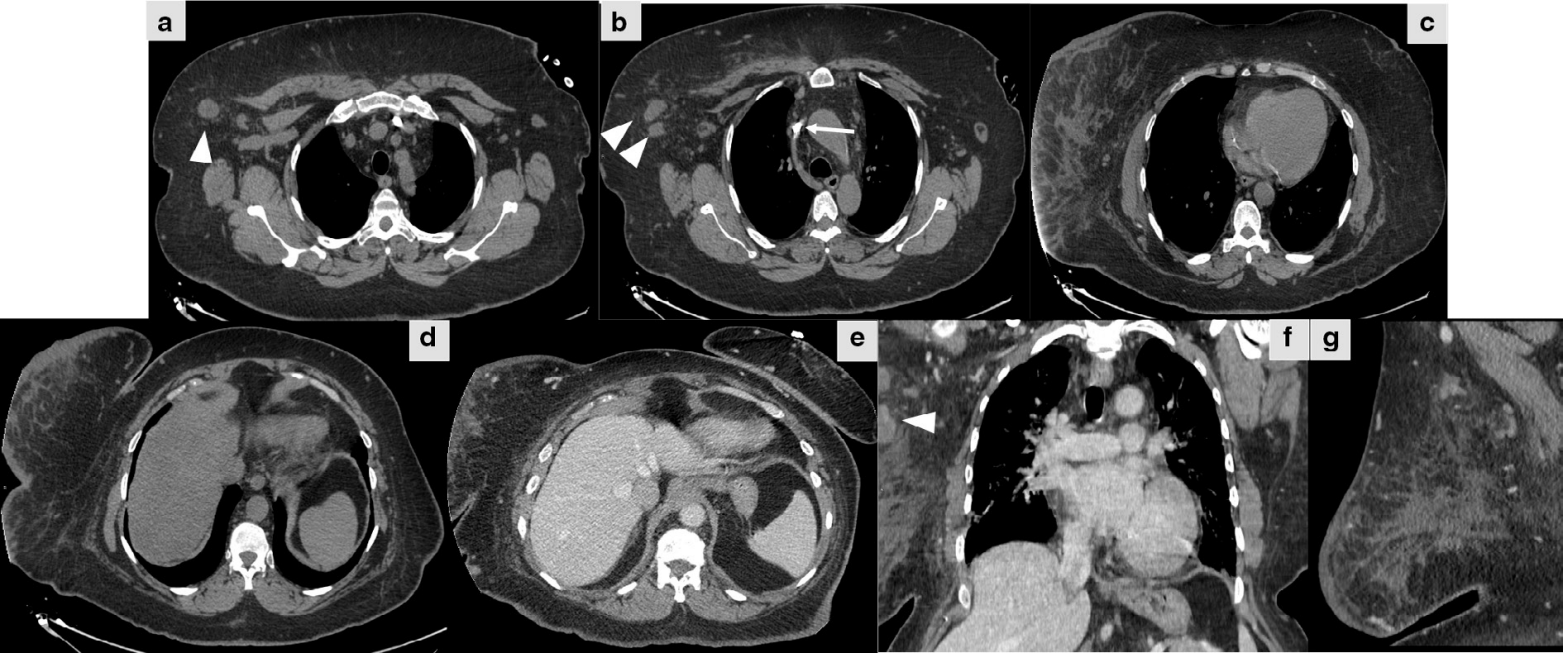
Non-enhanced CT scan of the chest, axial (a–e), coronal (f) and sagittal (g) images reveals a partially seen diffuse enlargement of the right breast with skin thickening and diffuse fat stranding and right multiple axillary lymphadenopathy (arrowheads). (e–g) Post-contrast CT coronal image shows no enhancement of the breast tissue. Partially seen Permacath inserted into left internal jugular vein (arrow) for heamodialysis.
